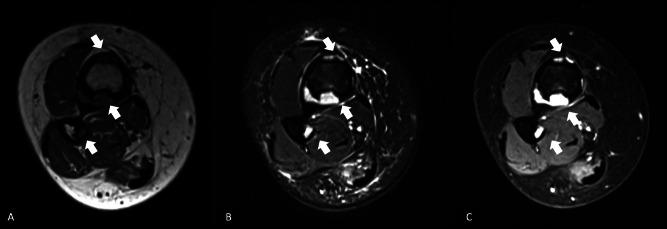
MRI of the distal right leg: T1‑weighted (A), T2 DIXON WATER (B), and post‑gadolinium contrast‑enhanced (C) sequences. Multiple intracortical lesions are centered in the cortex of the distal tibia, primarily involving the posterolateral and anterolateral aspects, with some extension into the anterior cortex (arrows). The lesions are hypointense on T1, hyperintense on T2 DIXON WATER, and show homogeneous enhancement after gadolinium administration. Additional small lesions are noted in the posteromedial cortex of the fibula.
